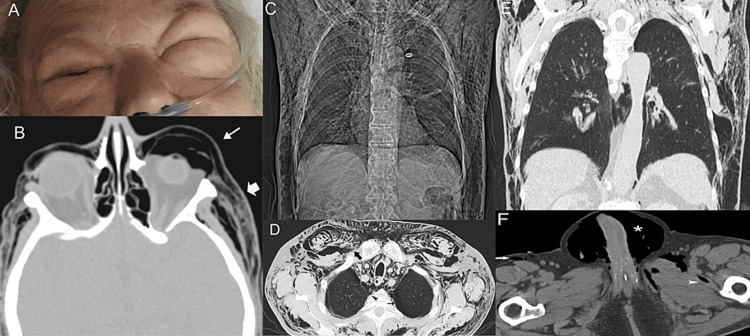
A 62-year-old man developed generalized pneumatosis after falling off a boat flagpole. Free air is seen literally from head to toe. A: A photograph of the patient shows facial pneumatosis; B: An axial CT image through the orbits shows emphysema of the upper lid (arrow) and the temporal region (thick arrow), on both sides; C: A coronal scout CT image shows marked pneumatosis occupying soft tissues of the lower neck, thorax, and upper abdomen; D: An axial CT image of the chest reveals pneumomediastinum (arrowhead), pneumothorax (arrow), and soft tissue emphysema (thick arrow); E: A coronal reformatted CT image of the chest shows pneumatosis; F: An axial CT image through the pelvis reveals marked scrotal emphysema (asterisk). Note free air in the subcutaneous soft tissue (arrow) and the intermuscular fascia (arrowhead).
